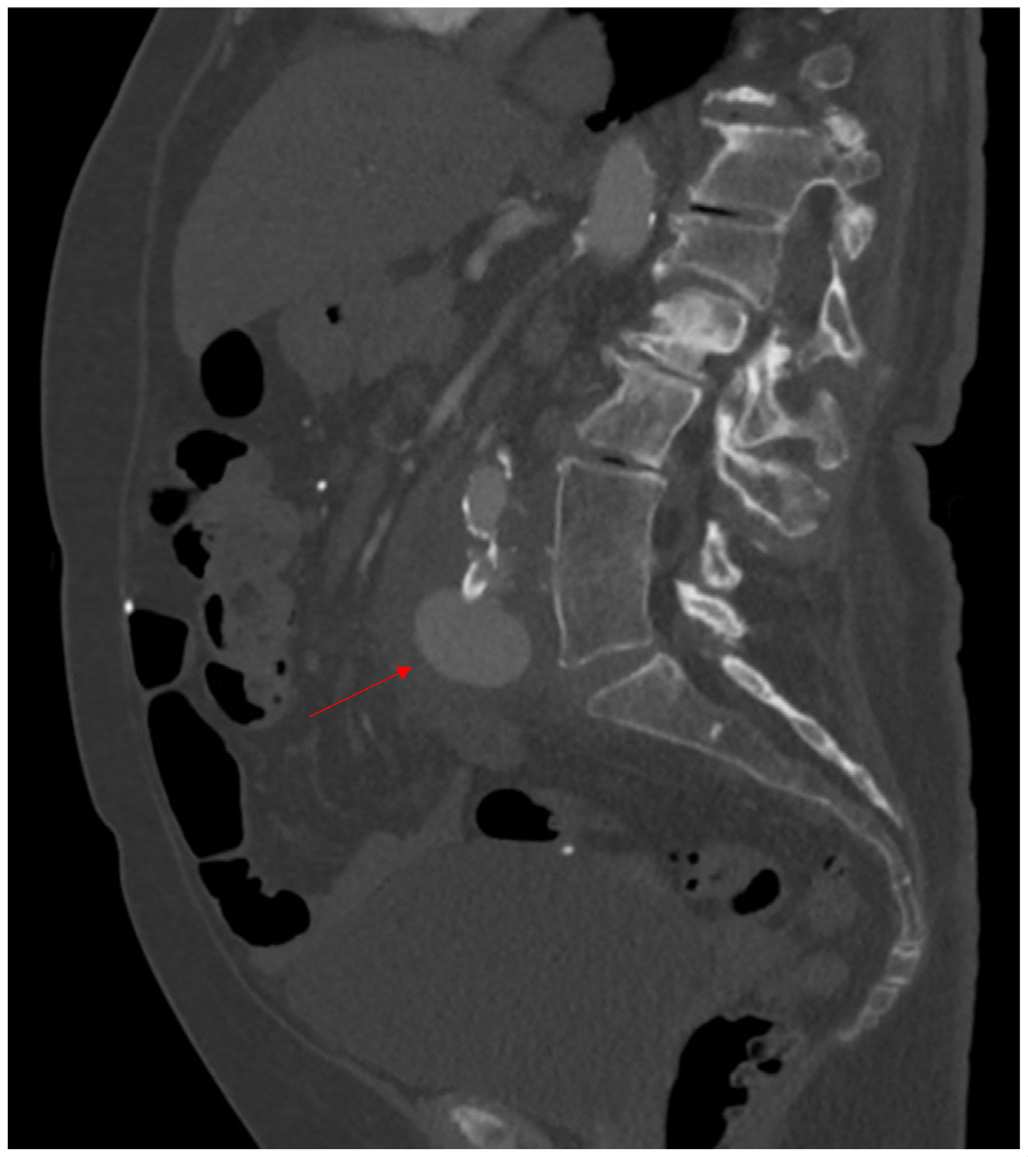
Computed tomography scan of right common iliac pseudoaneurysm (PSA), sagittal view (red arrow).
